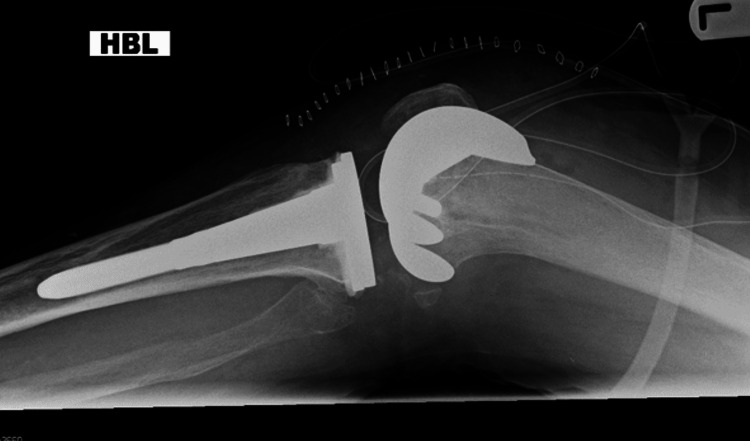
Radiograph with adequate gross lateral rotation
